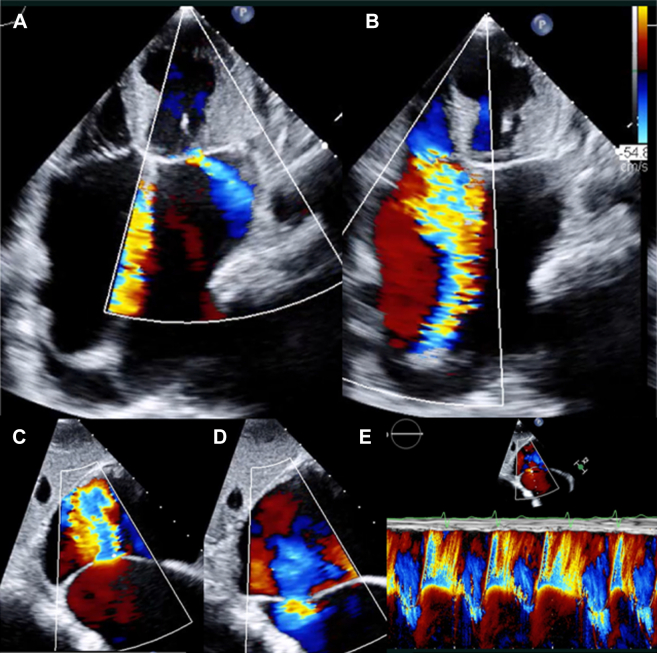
Acute Hemodynamic Collapse and Mechanical Ominous Sign Bedside transthoracic echocardiography during cardiogenic shock. (A) Successfully reduced mitral regurgitation (mild) but significantly exacerbated severe tricuspid regurgitation (TR). (B) High-velocity TR jet directly impinging on the interatrial septum. (C and D) Subcostal view revealing a large iatrogenic atrial septal defect with bidirectional shunting: (C) left-to-right and (D) right-to-left flow. (E) Color Doppler M-mode confirming the complex bidirectional shunt.
